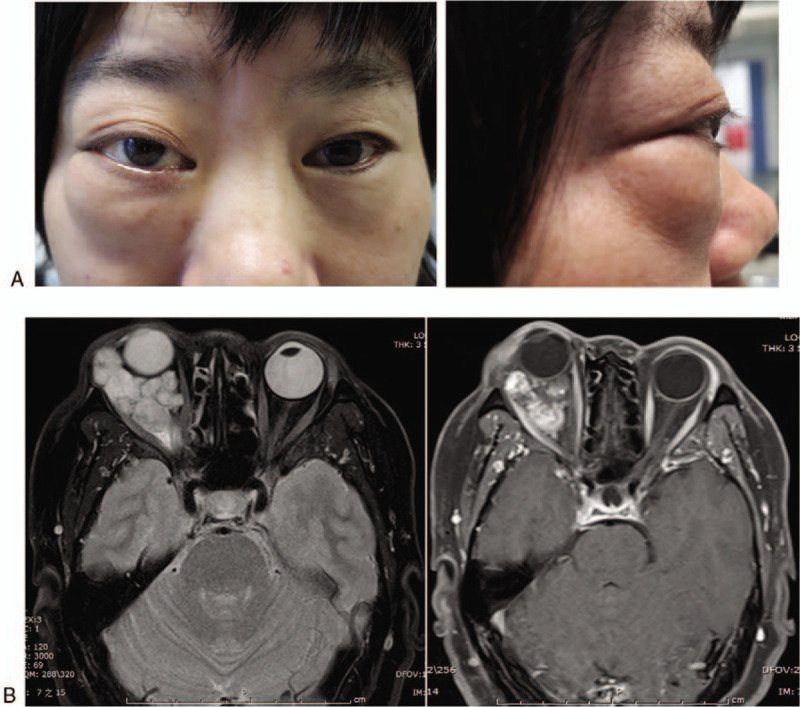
Ocular appearance and radiological examinations of the patient. (A) Front and lateral view of the patient. (B) T2-weighted magnetic resonance imaging showed a giant and irregular soft mass filling the right orbit up to the subcutaneous layer around the orbit, compressing the right optic nerve. T1-weighted image after gadolinium injection showed the heterogeneous and multi-focal enhancement signals in the masses.
